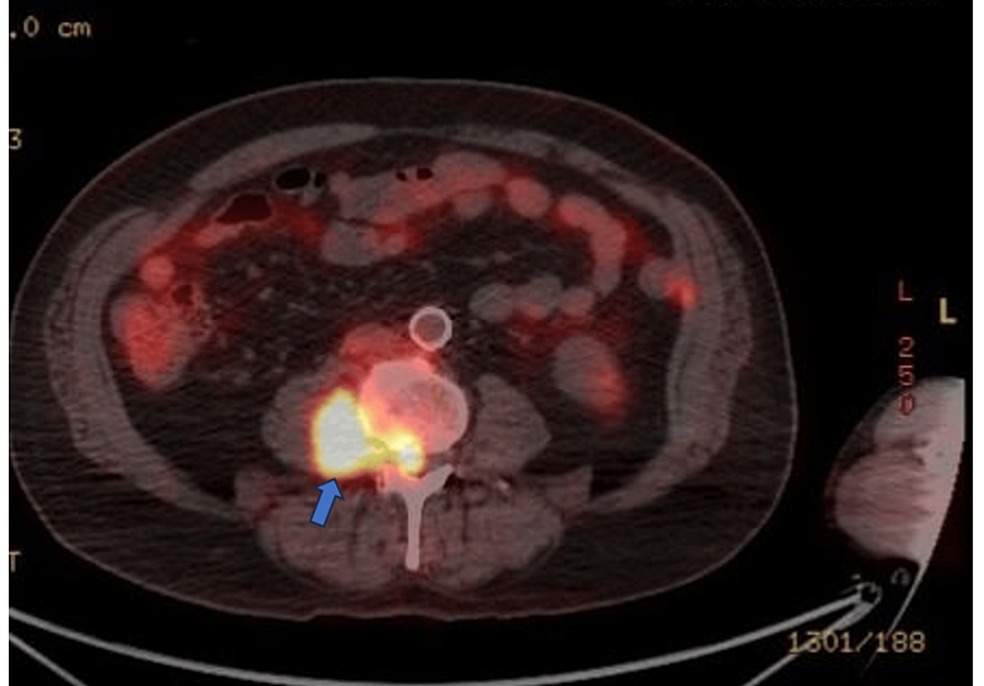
FDG avid paraspinal lesion extending to the spinal canal FDG: 18F-fluorodeoxyglucose
